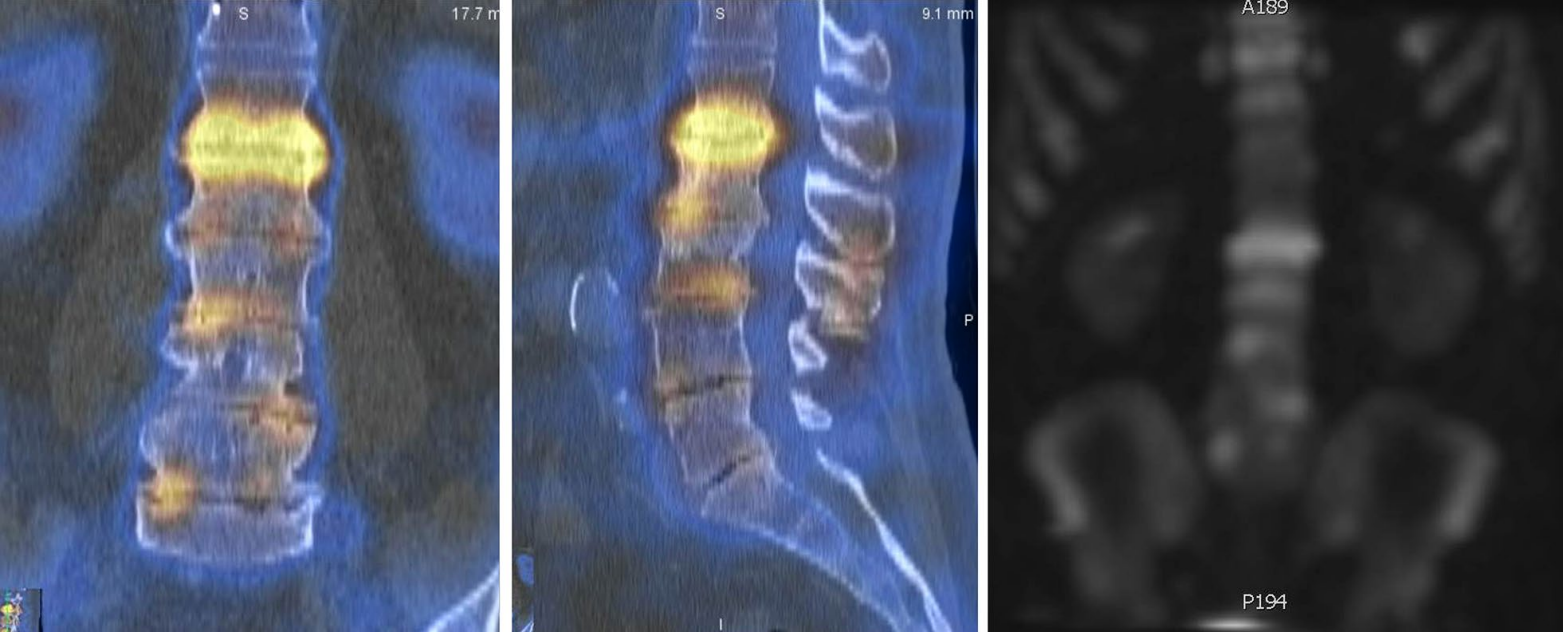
CT–SPECT of the lumbar spine. *Left* coronal and *center* sagittal merged images show increase Technetium uptake at the L1–2 level and minimal uptake at the other levels. *Right* the whole body bone scan (technique: 33.4 mCi of Technetium 99m MDP were administered intravenously. Bone scan images with SPECT imaging was obtained of the lumbar spine. These images were fused to CT lumbar spine of same date)
